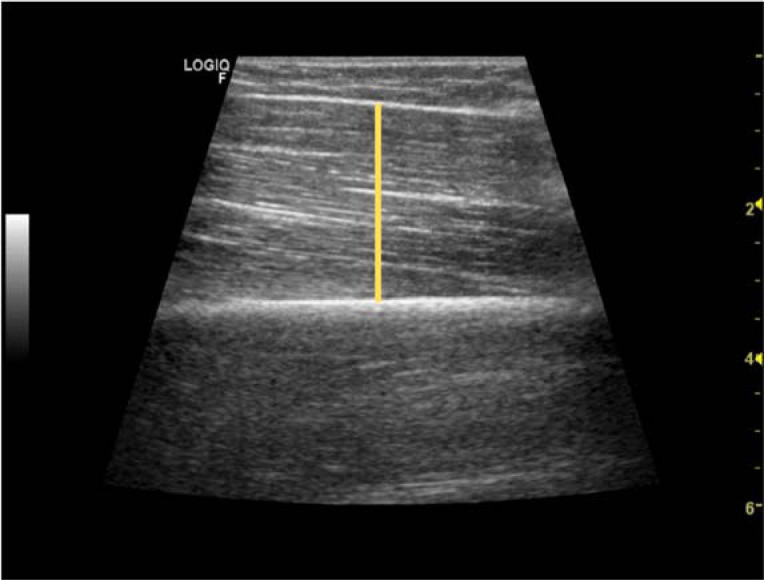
A representative measurement of MT of a subject from an Ultrasound Scan.
